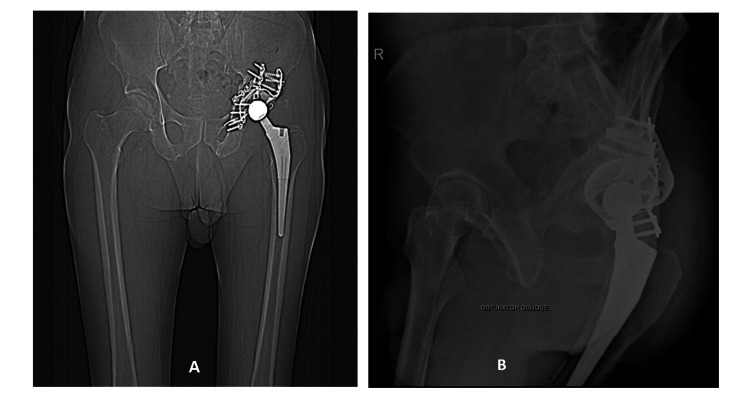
Five-year follow-up radiological imaging shows the implant in situ Panel A: Coronal view of CT scan showing the implant Panel B: Five-year follow-up X-ray of the pelvis with the implant
